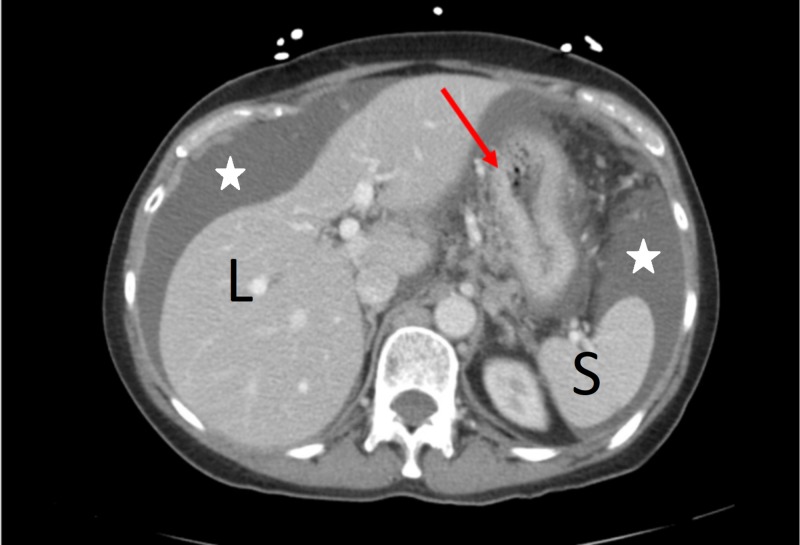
CT scan of the abdomen showing gastric wall thickening (red arrow) and ascites (white stars) L: liver; S: spleen
